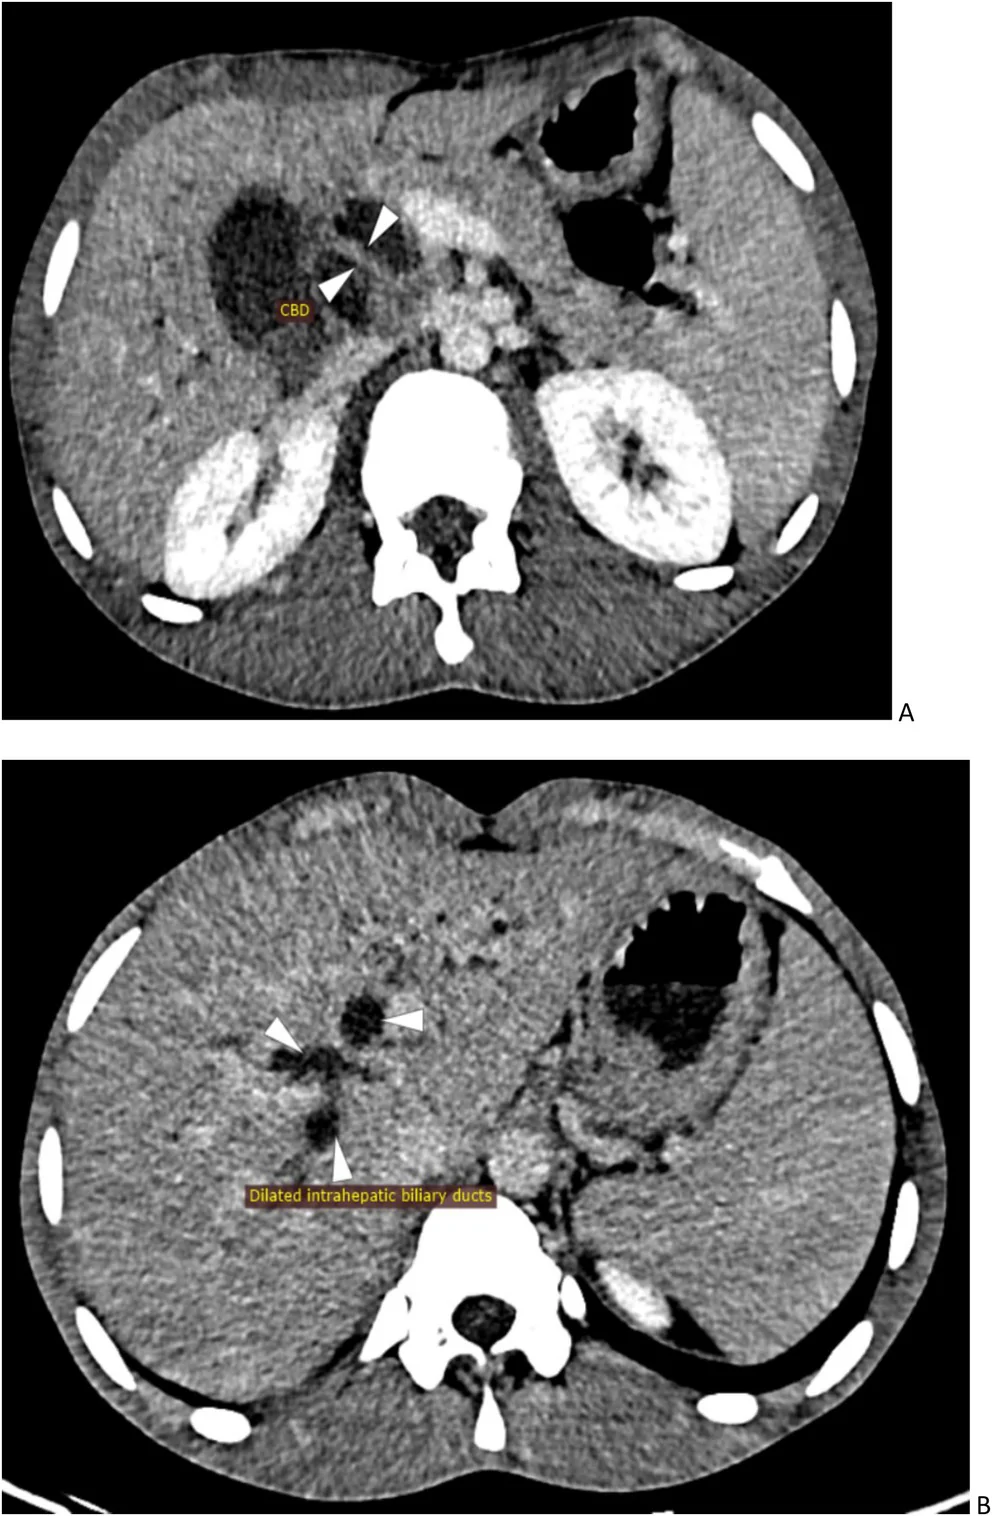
(A, B) Axial contrast‐enhanced CT image at the level of the common bile duct demonstrating narrowing of the mid common bile duct as it traverses the periportal lesion (A), resulting in upstream intrahepatic biliary duct dilatation (B).
